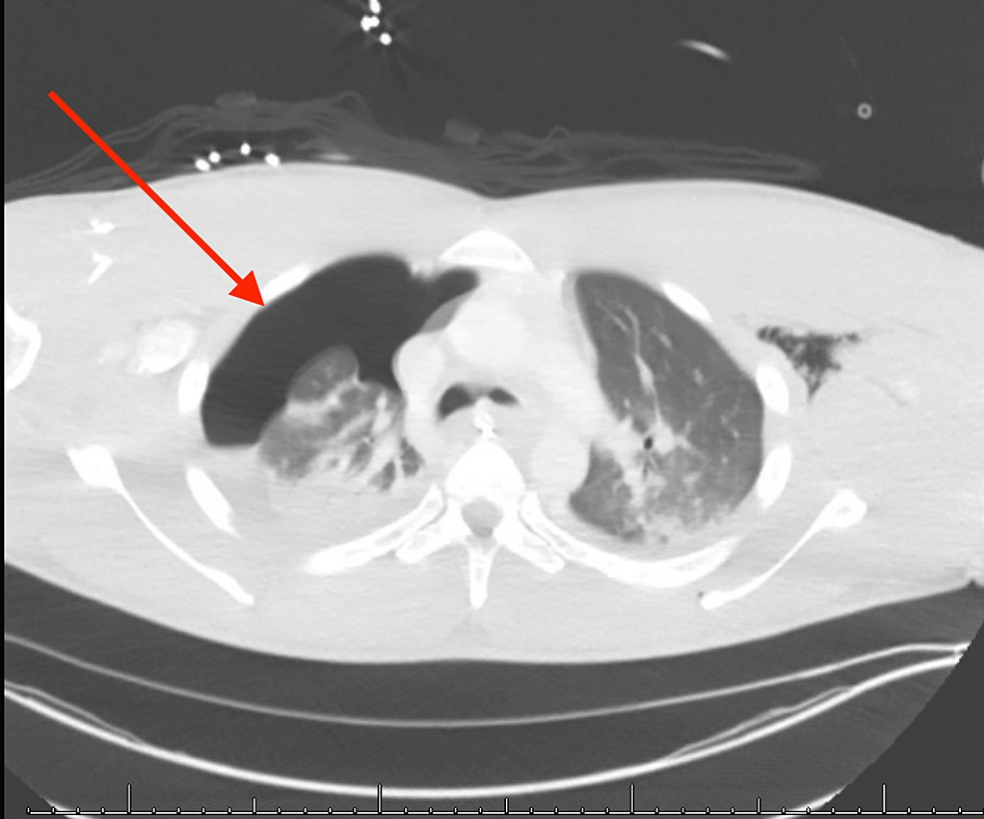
Large right pneumothorax on CT axial view of the thorax
